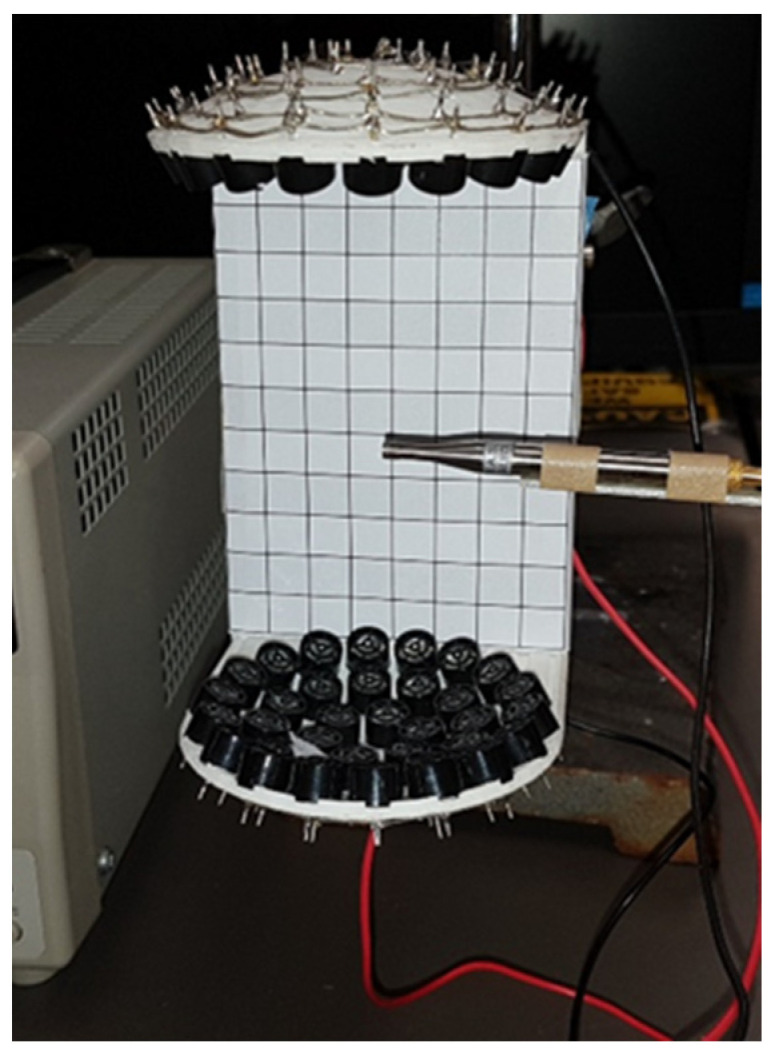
Ultrasonic array connected, and microphone inserted to test SPL.
